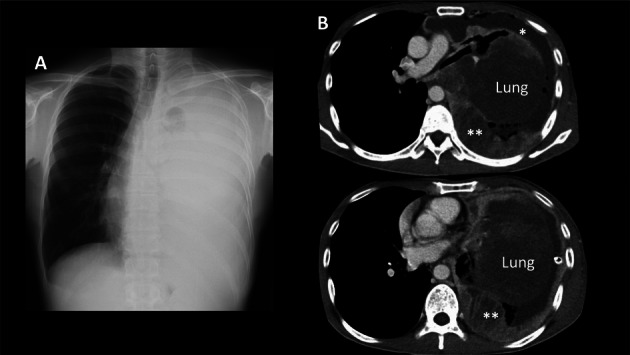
Chest X‐ray (A) and enhanced CT (B) at the time of the diagnosis, revealing massive left pleural effusion (*) and compressed lung, resulting in a rightward shift of the mediastinum. The left pleura was irregularly thickened on the left side of the back (). Enlarged lymph nodes or distal metastases were not observed. CT, computed tomography.
